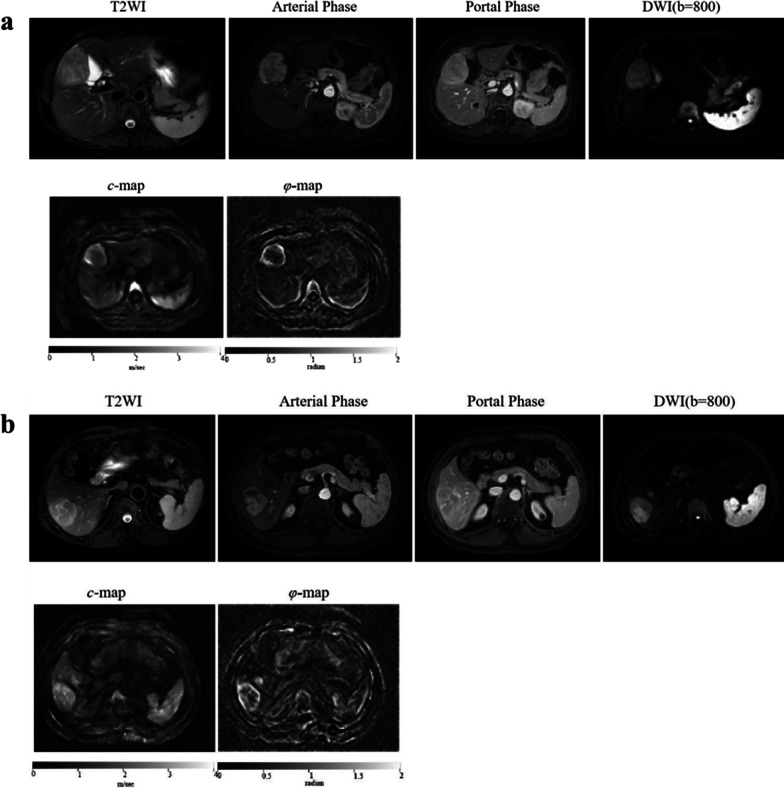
Representative patients’ images. Axial T2-weighted images, arterial-phase images, portal-phase images, diffusion-weighted images at a b value of 800 s/mm^2^, axial *c* and *φ* maps of tumors obtained in (a) a patient with proliferative HCC (tumor *c*: 2.31 m/s, tumor *φ*: 1.23 rad, liver *c*: 1.91 m/s, and liver *φ*: 0.68 rad) and (b) a patient with non-proliferative HCC (tumor *c*: 2.51 m/s, tumor *φ*: 1.11 rad, liver *c*: 1.65 m/s, and liver *φ*: 0.79 rad)
